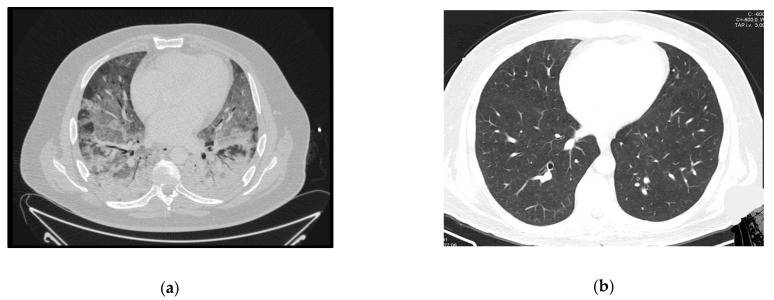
Case 4 thoracic CT-scan at diagnosis (a) and follow-up (b).
